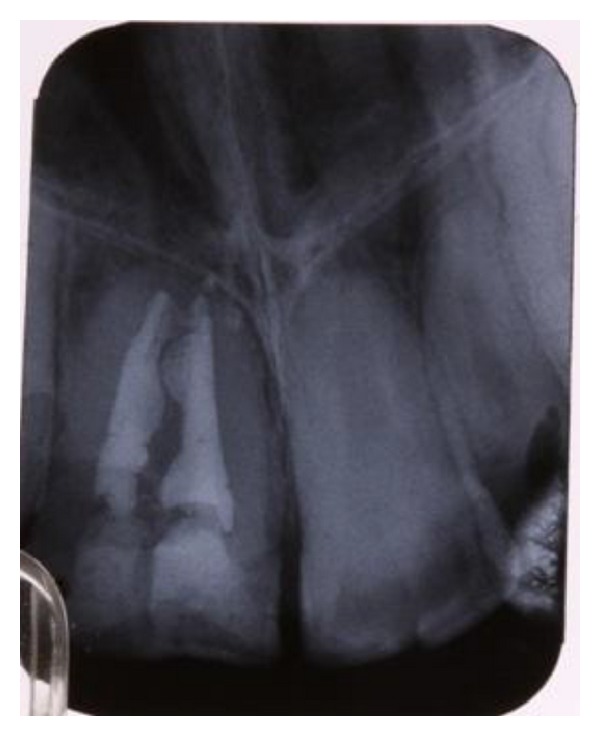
Periapical view of the treated canal.
